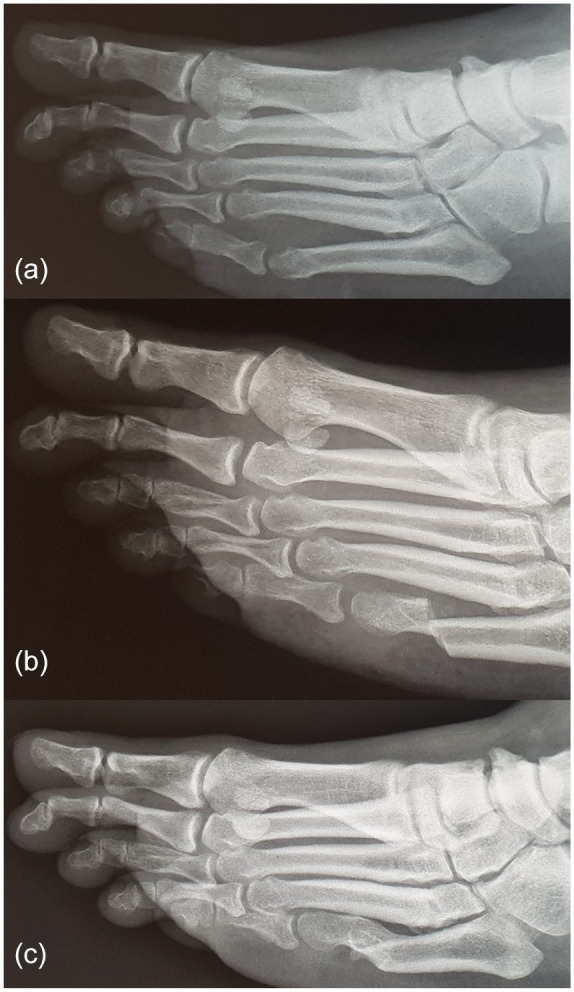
Radiographs of fifth metatarsal: (a) presurgery; (b) 1 week postsurgery; (c) 2 years postsurgery.
